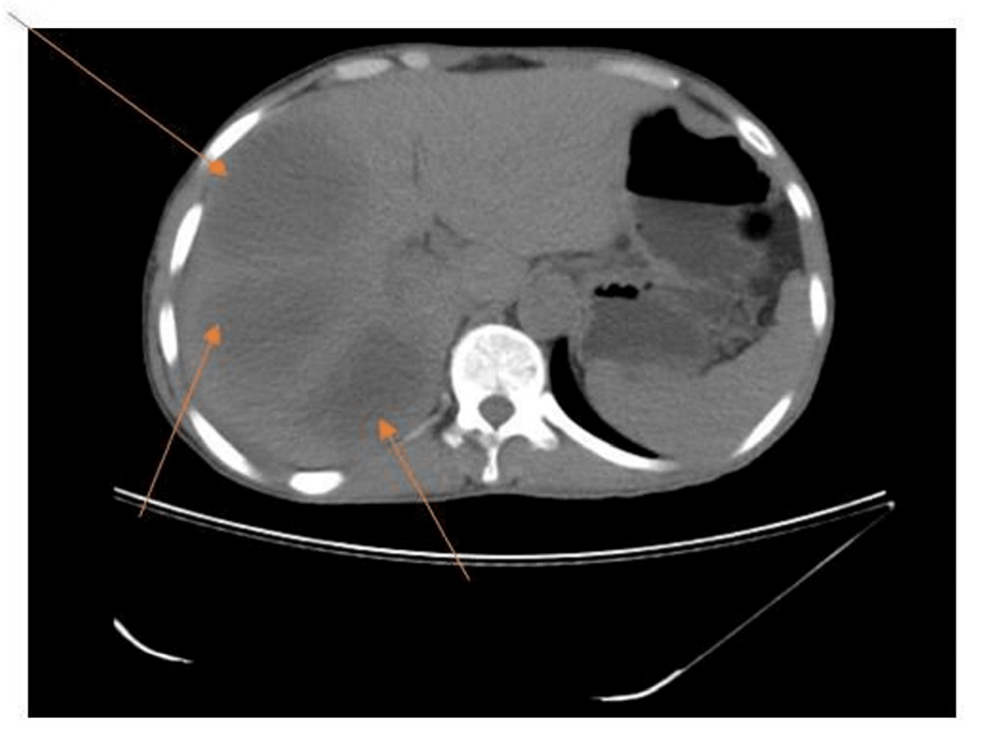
Repeat CT scan with IV contrast The image shows Hepatomegaly with large, thick-walled, peripherally enhancing collections showing few air pockets within the segments V, VI, VII, and VIII of the right lobe of the liver, only mildly reduced as compared with the previous collection (as shown by the arrows) CT: computed tomography
